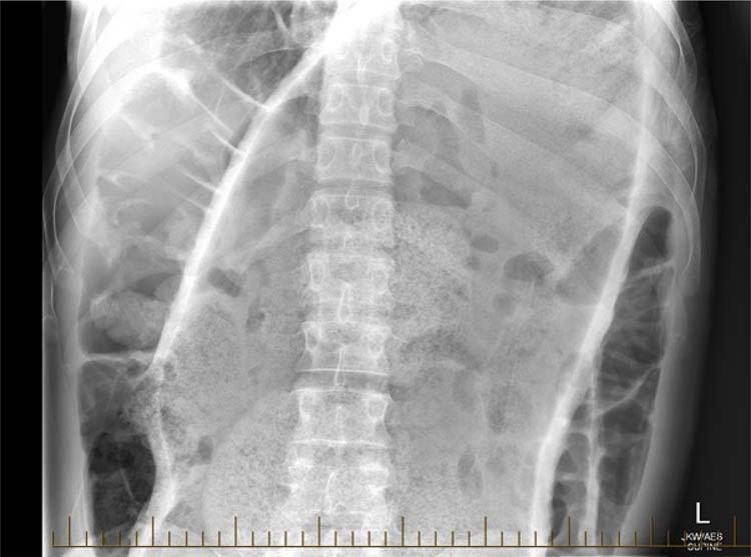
Plain film radiograph of the abdomen.
